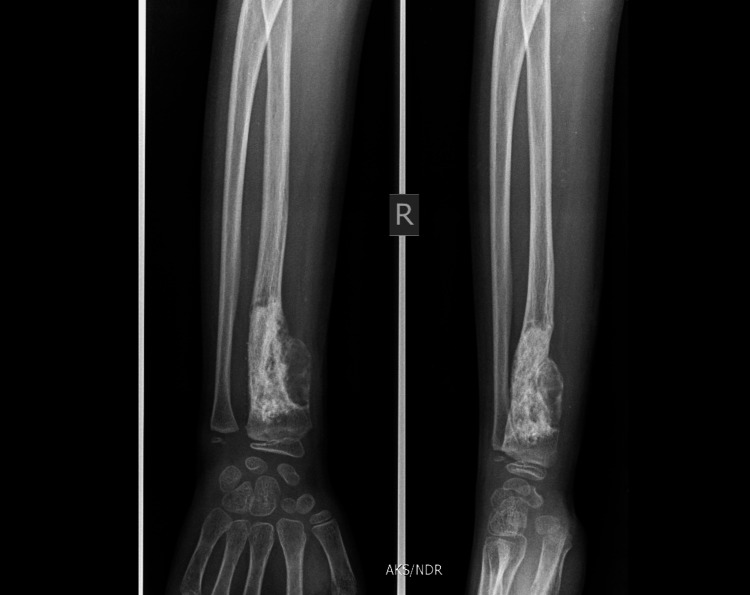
Check X-ray at the end of six months showing a good integration of the graft with the parent bone with obliteration of the cavity
